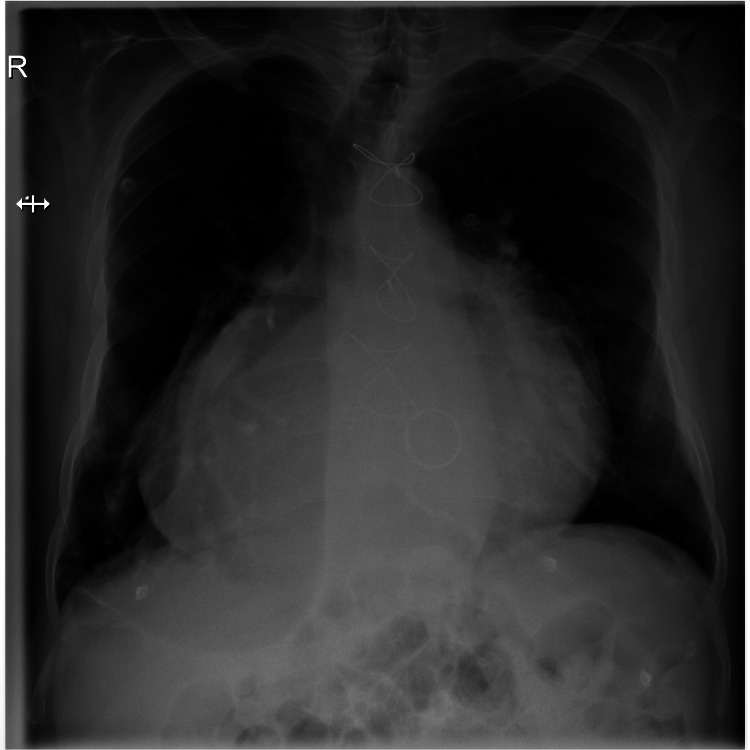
Chest X-ray showing massive cardiomegaly
